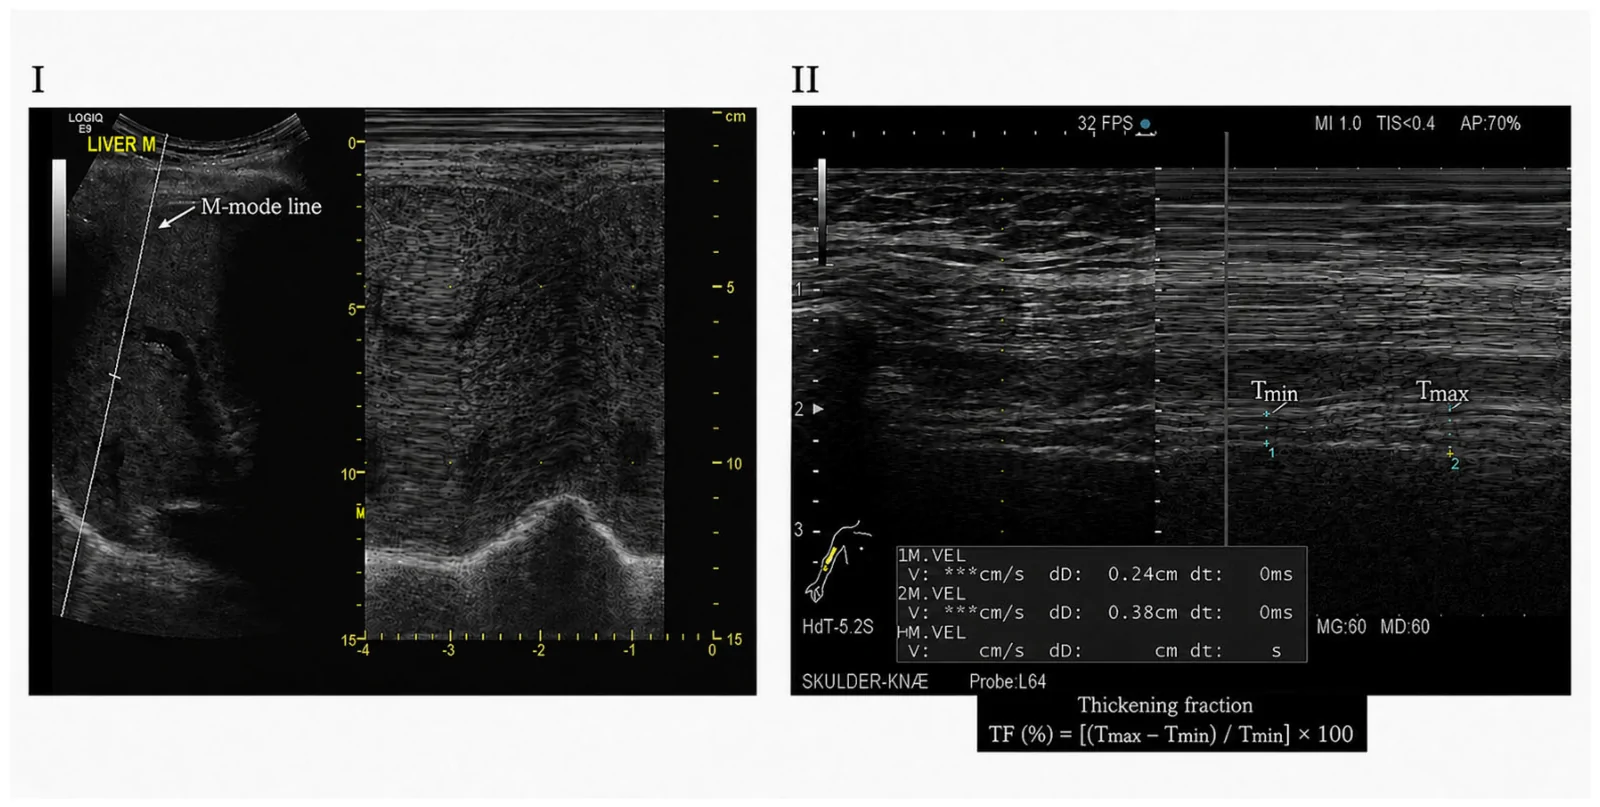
Different types of diaphragmatic ultrasound measurements. (I) B-mode image showing placement of the M-mode sampling line across the diaphragm, with the corresponding M-mode trace used to assess diaphragmatic excursion. (II) M-mode assessment of diaphragm thickness at minimum thickness and maximum thickness. Thickening fraction (TF) was calculated as: TF (%) = [(Tmax − Tmin)/Tmin] × 100.
